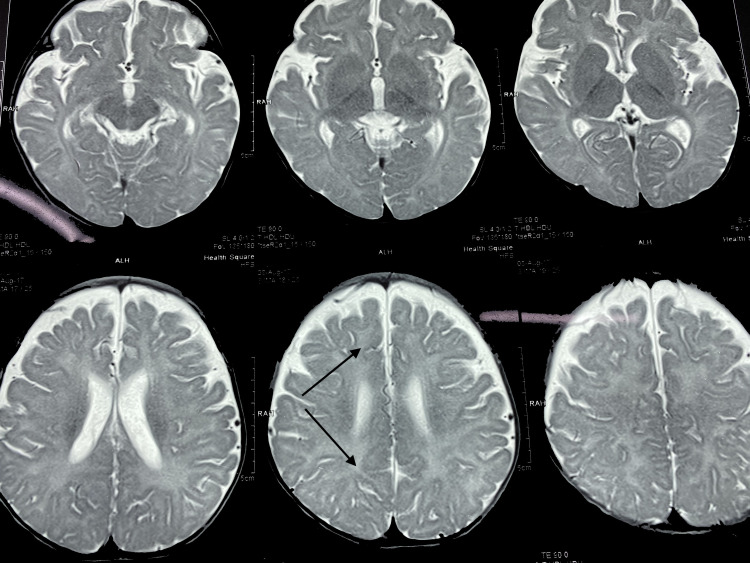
Hypomyelination of deep and subcortical white matter on T2-weighted images
